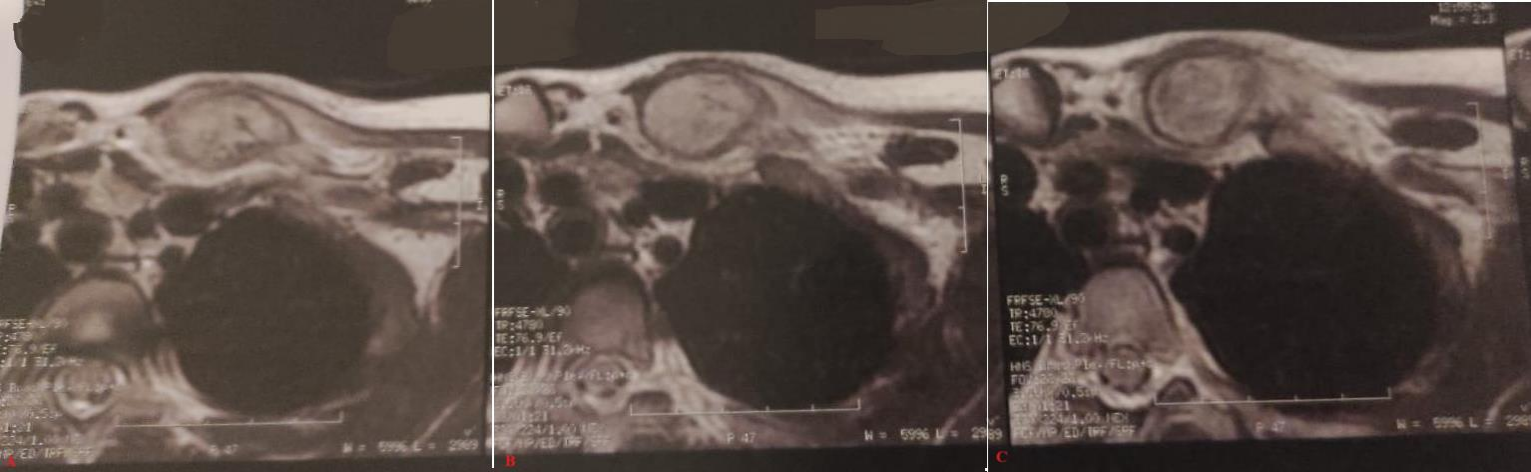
A,B,C) clavicle magnetic resonance imaging: axial section; low intense lesion in T1-weighted images and high intense in T2-weighted, heterogeneously enhancing, edematous signal of the surrounding soft
